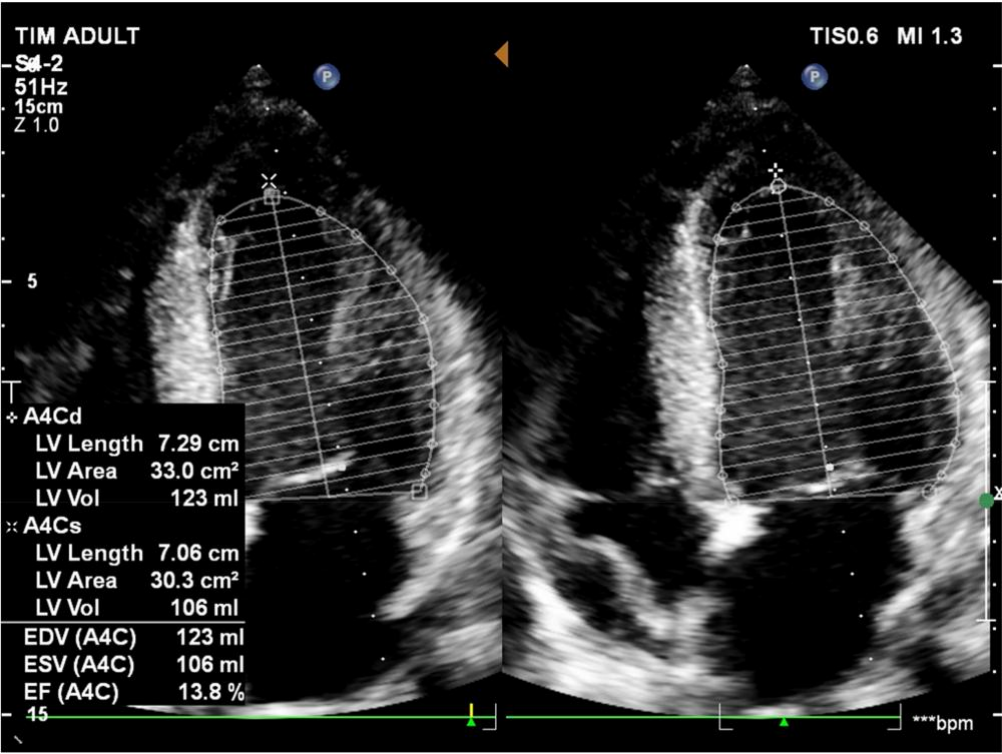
Echocardiography revealed a global reduction in left ventricular function, with an ejection fraction (EF) of 14%.
